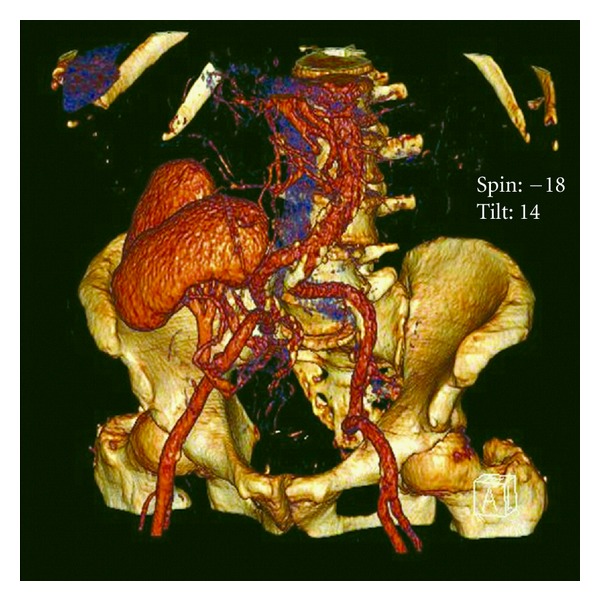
CT 3D reconstruction of the monolateral dual kidney transplantation implanted on the right iliac vessels.
